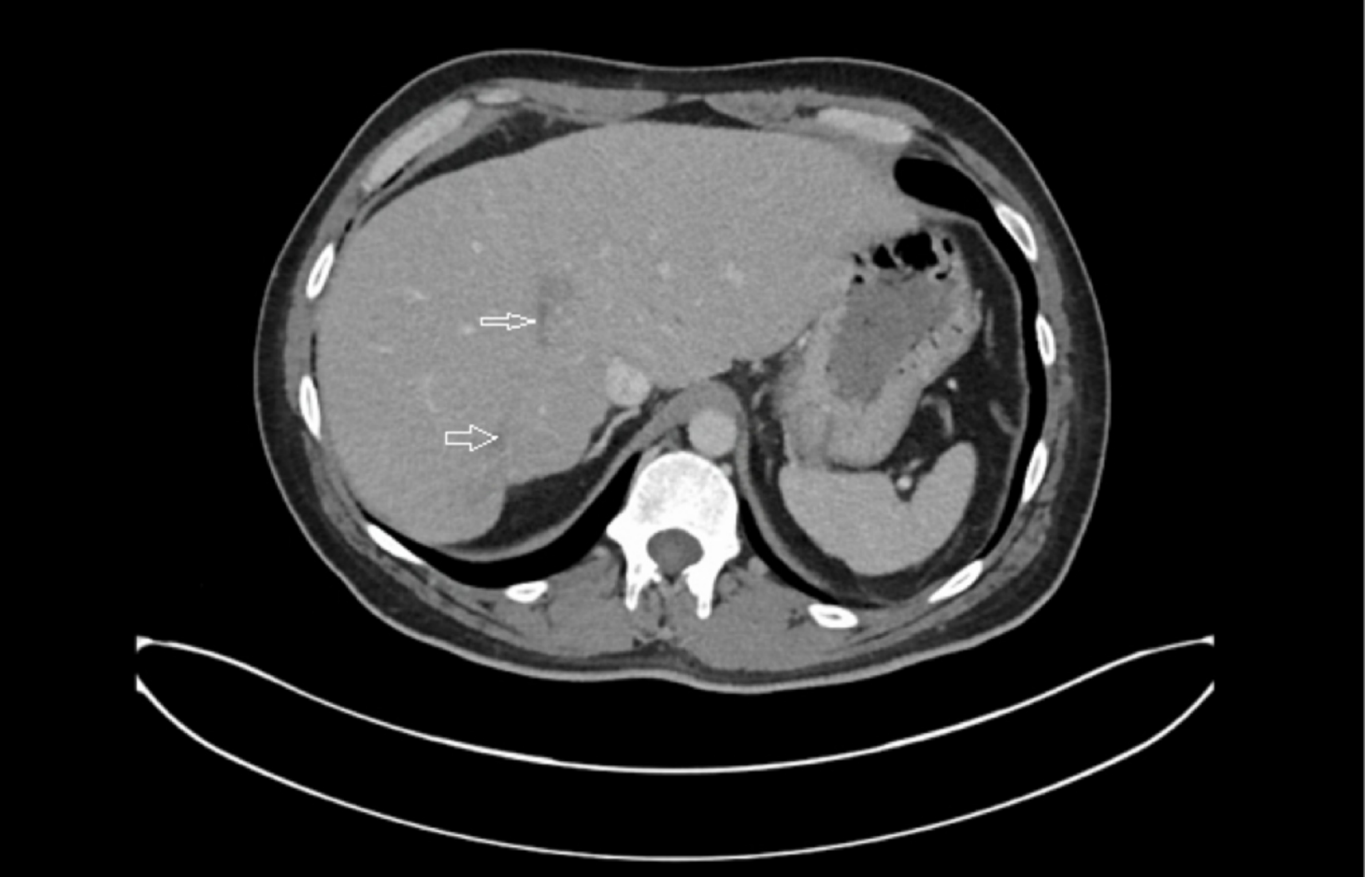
CT abdomen done in December 2019. Depicting resolution of both liver abscesses after percutaneous drainage and six weeks of IV antibiotics.
